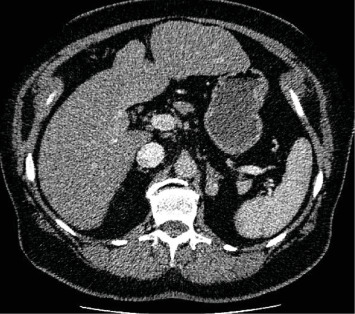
Abdominal CT scan demonstrating the adrenal adenoma of the left adrenal gland in a patient, with mild autonomous cortisol production and related comorbidities (case 1).
